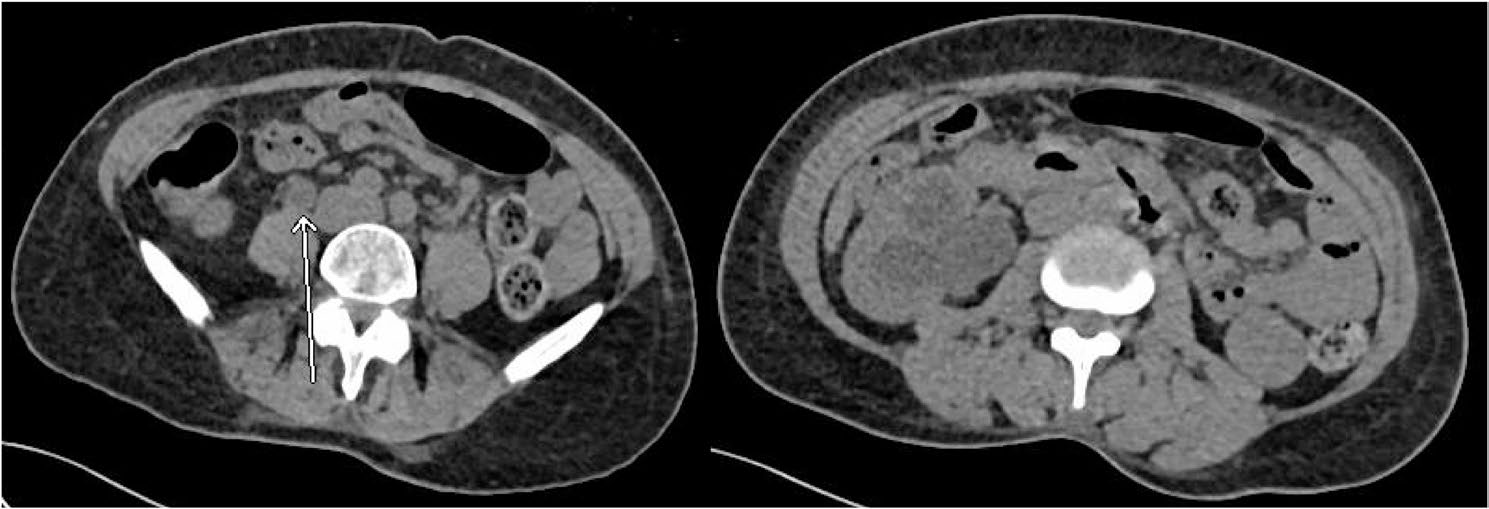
CT scan showing obstructive uropathy with hydronephrosis
